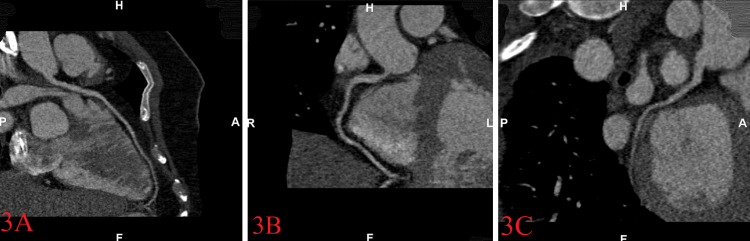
Coronary CT angiography showing the coronary arteries arise in normal positions with no evidence of coronary stenosis or plaque in the left anterior descending (A), right coronary (B), and left circumflex arteries (C). Total calcium score of zero indicating the absence of calcified plaques in the coronary tree. Labels: H = Head; F = Feet; P = Posterior; A = Anterior; R = Right; L = Left
